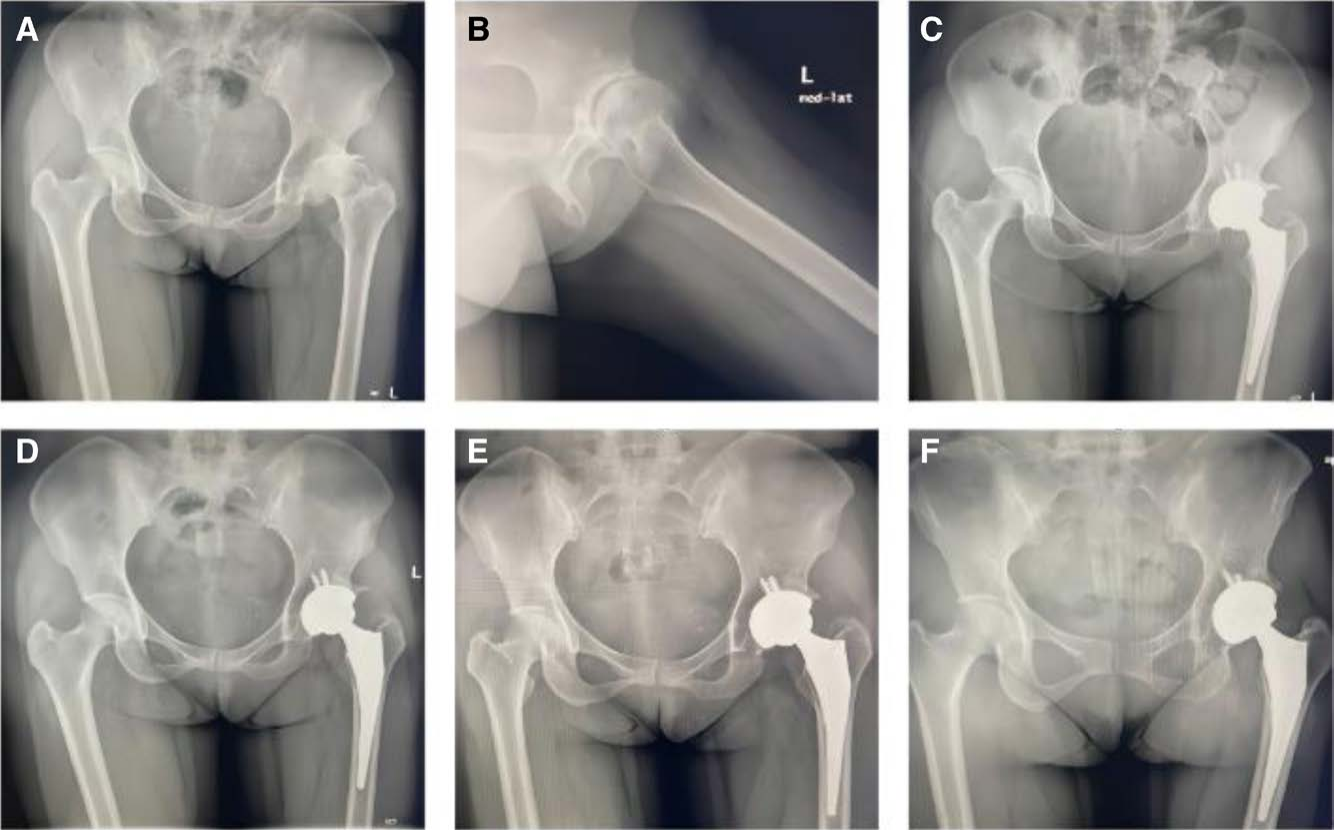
Direct anterior approach total hip arthroplasty for a 36-yr-old female patient. (A) Preoperative pelvis X-ray. (B) Preoperative hip X-ray. (C) Postoperative pelvis X-ray 1 wk after surgery. (D) Postoperative pelvic X-ray 3 mo after surgery. (E) Postoperative pelvic X-ray 6 mo after surgery. (F) Postoperative pelvic anteroposterior X-ray 1 yr after surgery.
